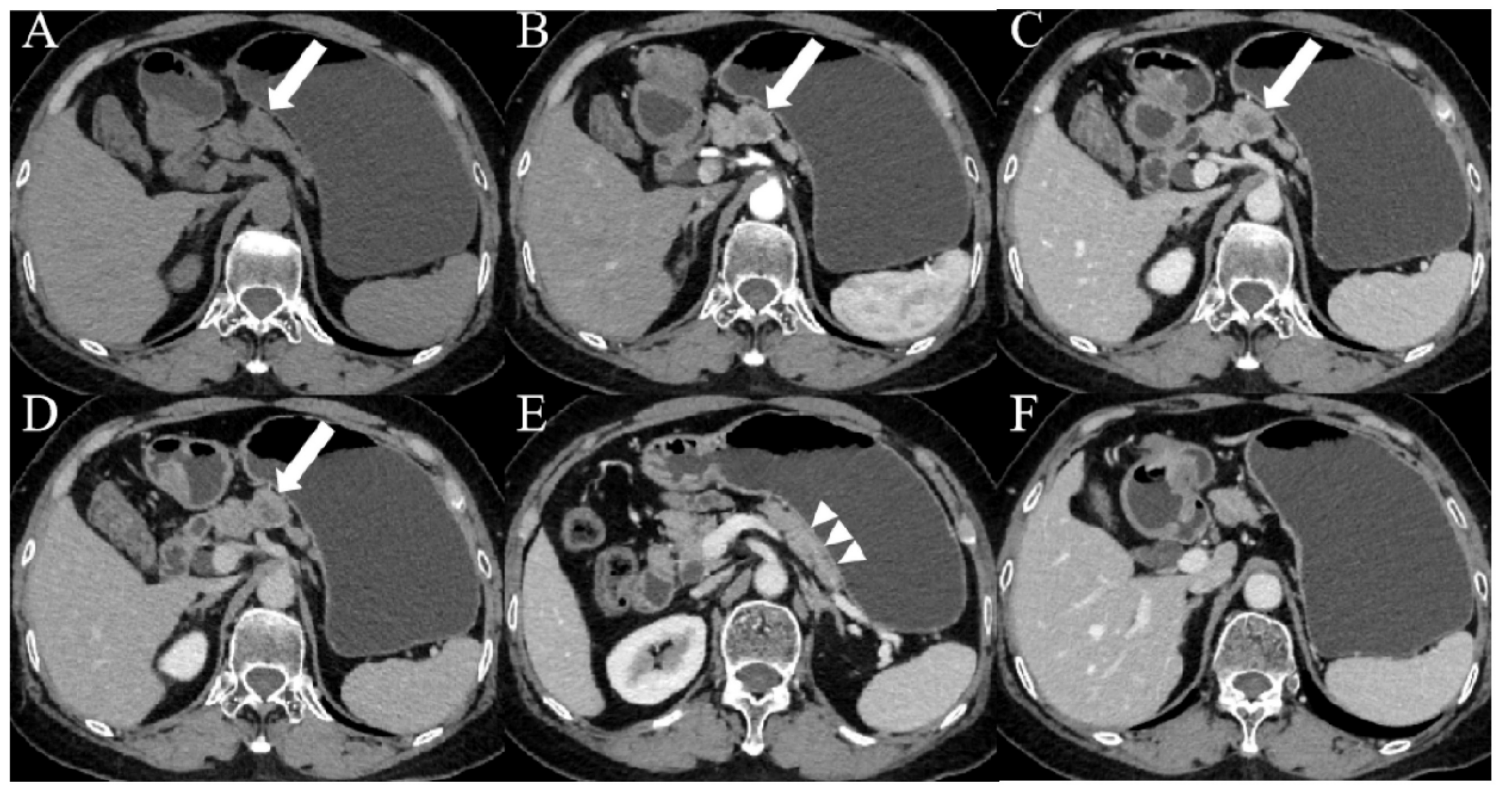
Images from a patient with pNET G2. (A-D) CT images showed a nodular, slightly enhanced area (white arrows) in the body of the pancreas, with internal cystic non-enhancing regions and an indistinct margin. (E) CT imaging showed a normal pancreatic tail without atrophy (arrowheads). (F) The fat spaces around the pancreas were clear, with no markedly enlarged lymph nodes. The patient's CA19-9 level was 19.6 U/mL (< 37 U/mL), and the D-dimer level was 0.23 mg/L (< 0.84 mg/L).
